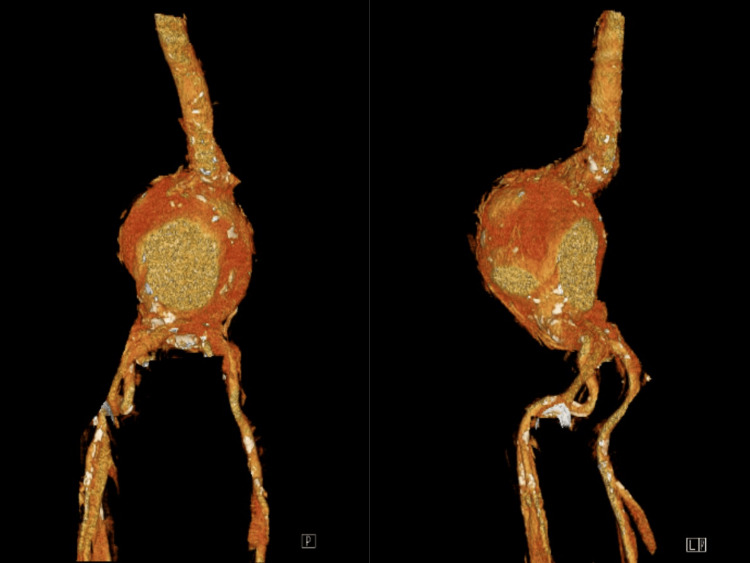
Contrast-enhanced 3D CTA of a giant infrarenal AAA 3D CTA showing a giant infrarenal abdominal aortic aneurysm (134 × 121 × 124 mm) with eccentric mural thrombus and a reduced central lumen, extending to the aortic bifurcation with involvement of both common iliac arteries. AAA, abdominal aortic aneurysm; CTA, CT angiography
